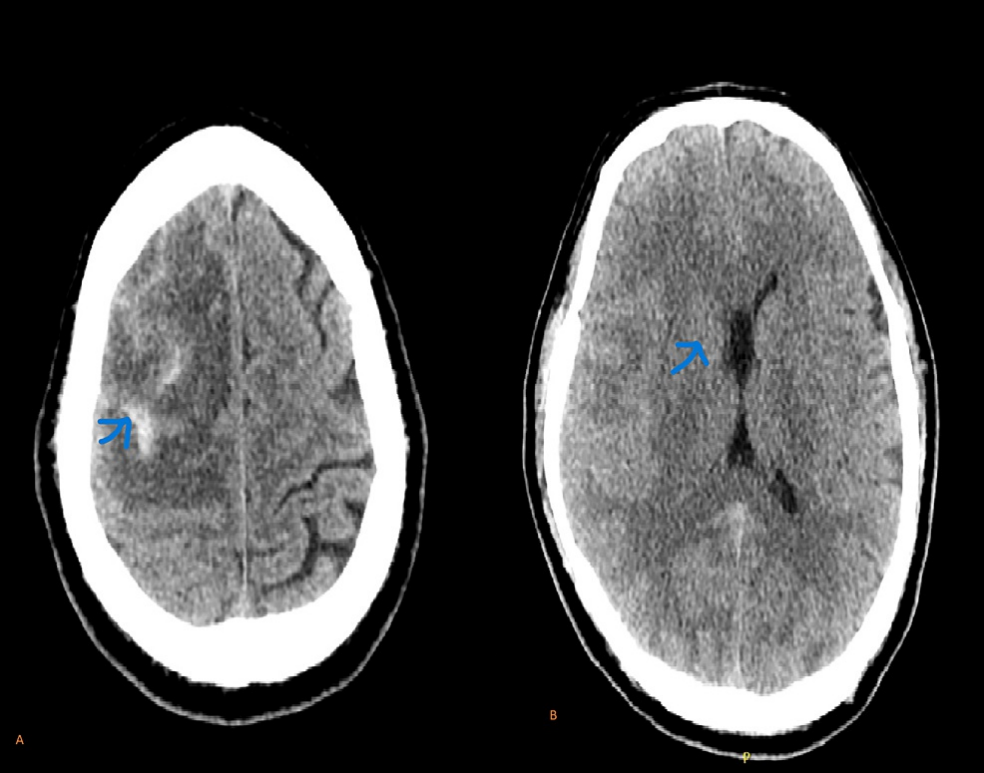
CT scan of the brain in the axial view (A, B) A: Acute right frontal subarachnoid hemorrhage with perifocal edema (arrow) B: Mass effect on the frontal horn of right lateral ventricle (arrow)
